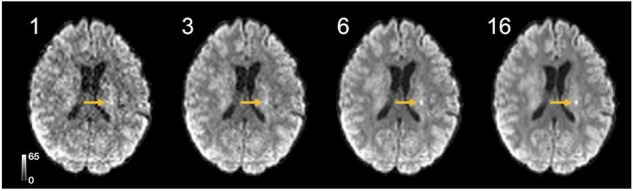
High-quality high *b*-value STE-DWI (*b* = 2,000 s/mm^2^) images can be obtained with short scan times and few averages. The tumor-related hyperintensity is visible with 3 averages (yellow arrows), but becomes clearer with 6 averages and yields an image quality similar to that obtained with 16 averages. With a repetition time of 5,000 ms, 6 averages can be acquired in 30 s.
